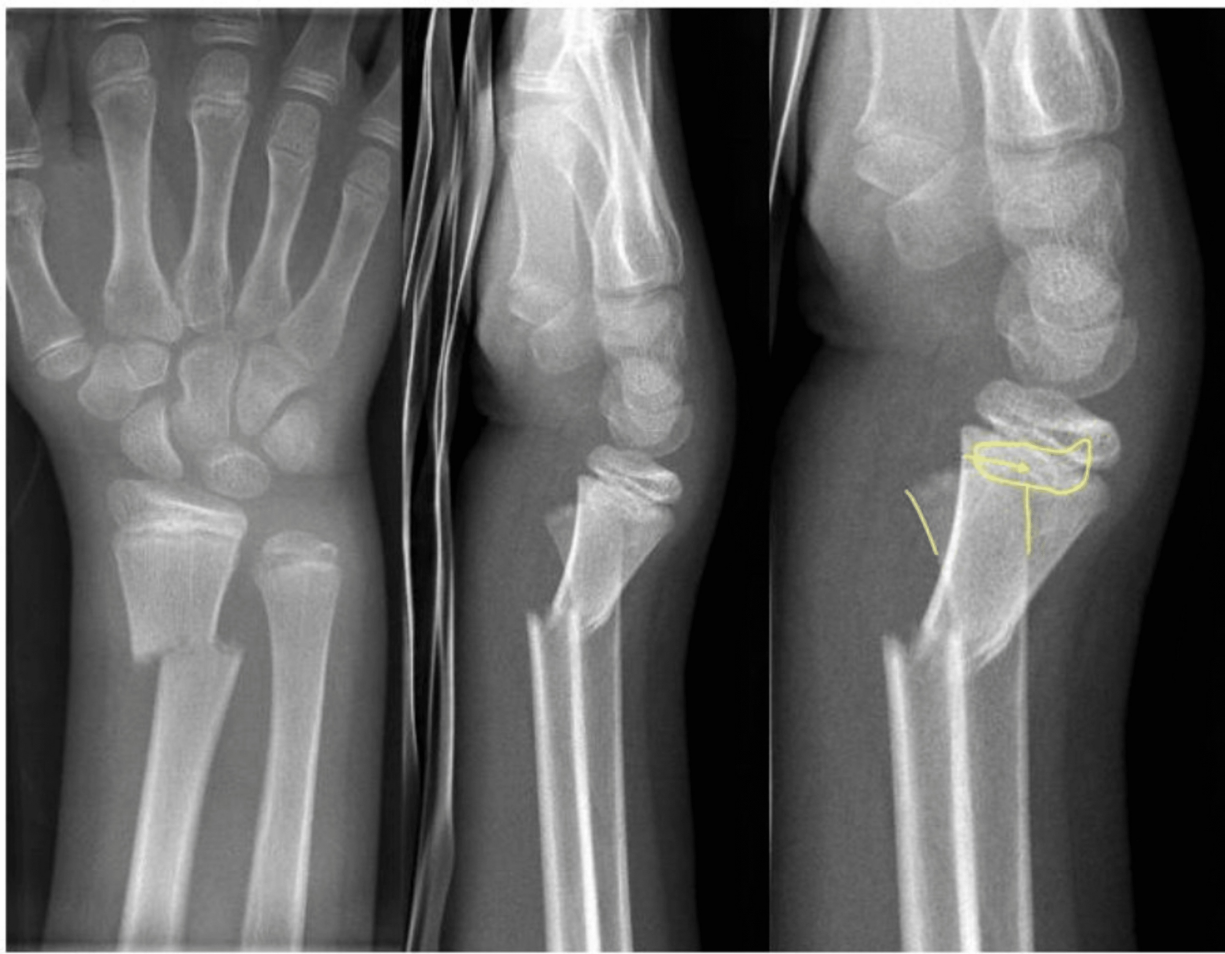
X-rays before surgery X-rays (anteroposterior and lateral view) with distal radial fracture and disruption of the distal ulnar physis. Yellow: epi-metaphyseal relation.
